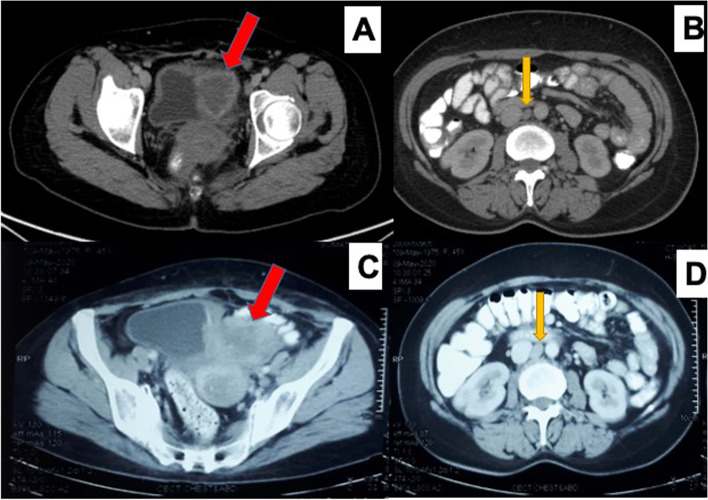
A Axial computed tomography (CT) scan images at baseline showing a large proliferative growth involving left lateral wall of urinary bladder with perivesical extension and vesicoureteric junction involvement (red arrow). B The same CT image showed multiple enlarged pelvic and paraaortic and aortocaval lymph nodes (yellow arrow) which were 18F-fluorodeoxyglucose (FDG) avid on corresponding positron emission tomography (PET) image. C Contrast-enhanced CT following two lines of chemotherapy showing progression in bladder growth (red arrow), while the paraaortic nodes were unchanged (yellow arrow) but still enhancing (D)
